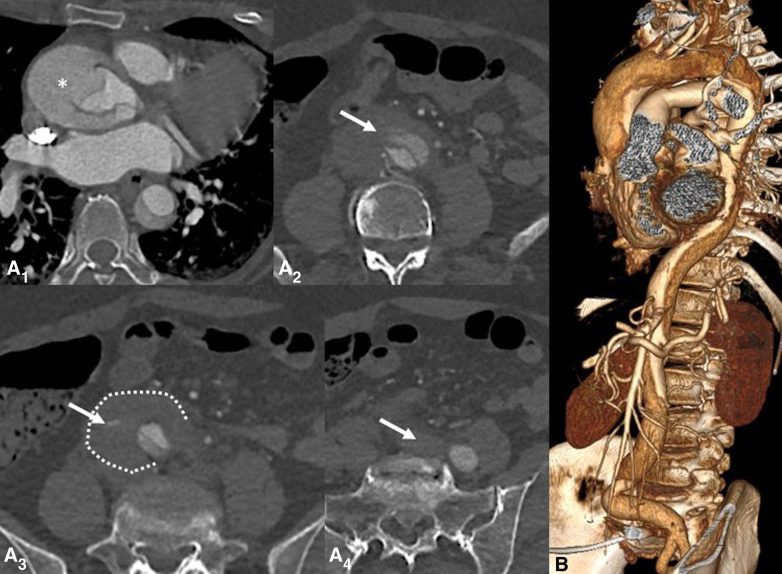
Preoperative computed tomography angiography showing acute type A aortic dissection (A_1_) (*asterisk*) with the infrarenal rupture (A_2_) (*white arrow*) that was contained with a recent bleeding inside (A_3_) (*white arrow*) the retroperitoneal hematoma (A_3_) (*dotted line*). Peripheral malperfusion was caused by static obstruction of the right common iliac artery (A_4_) (*white arrow*). Three-dimensional volume rendering reconstruction of the Debakey type 1 dissection (B).
